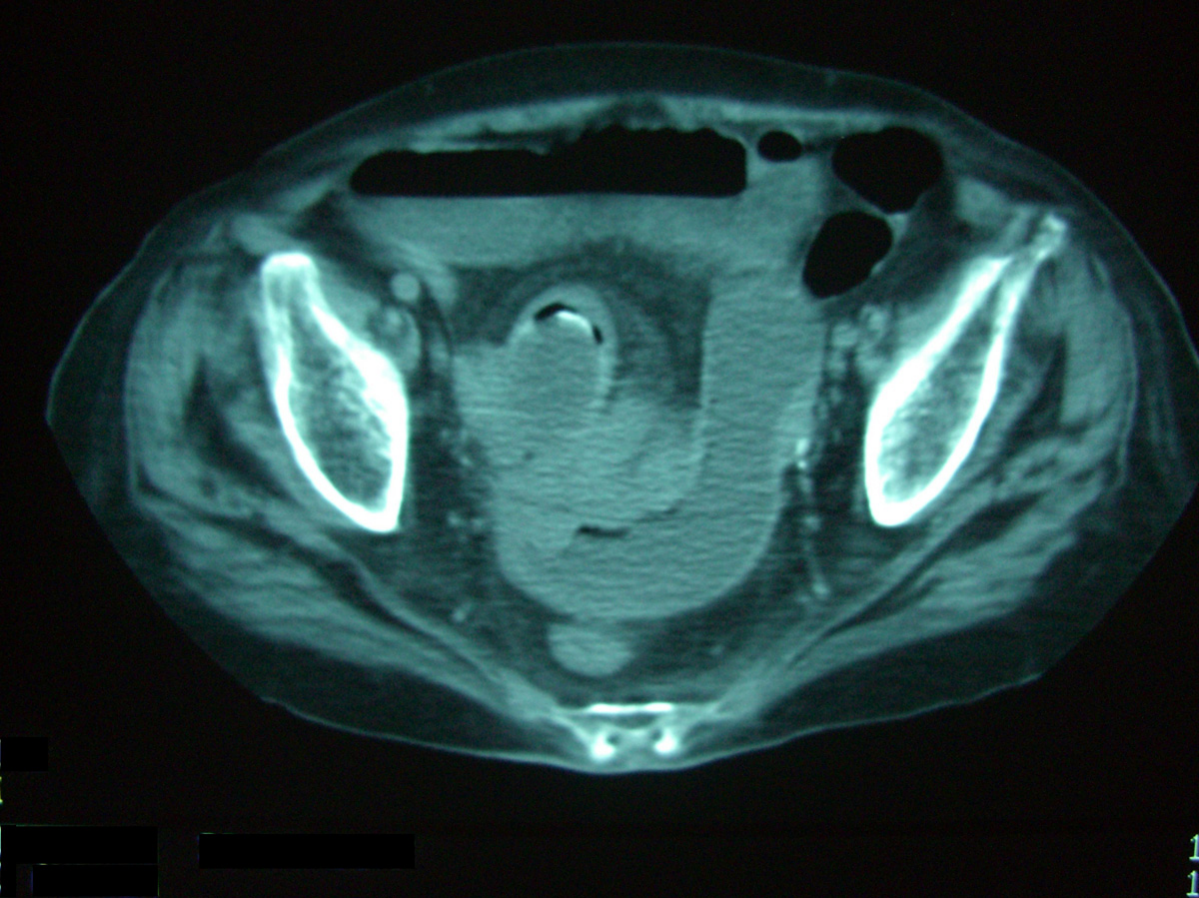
CT scan case 2: Intestin loops distention, with air-fluid levels and endoluminal gallstone.
